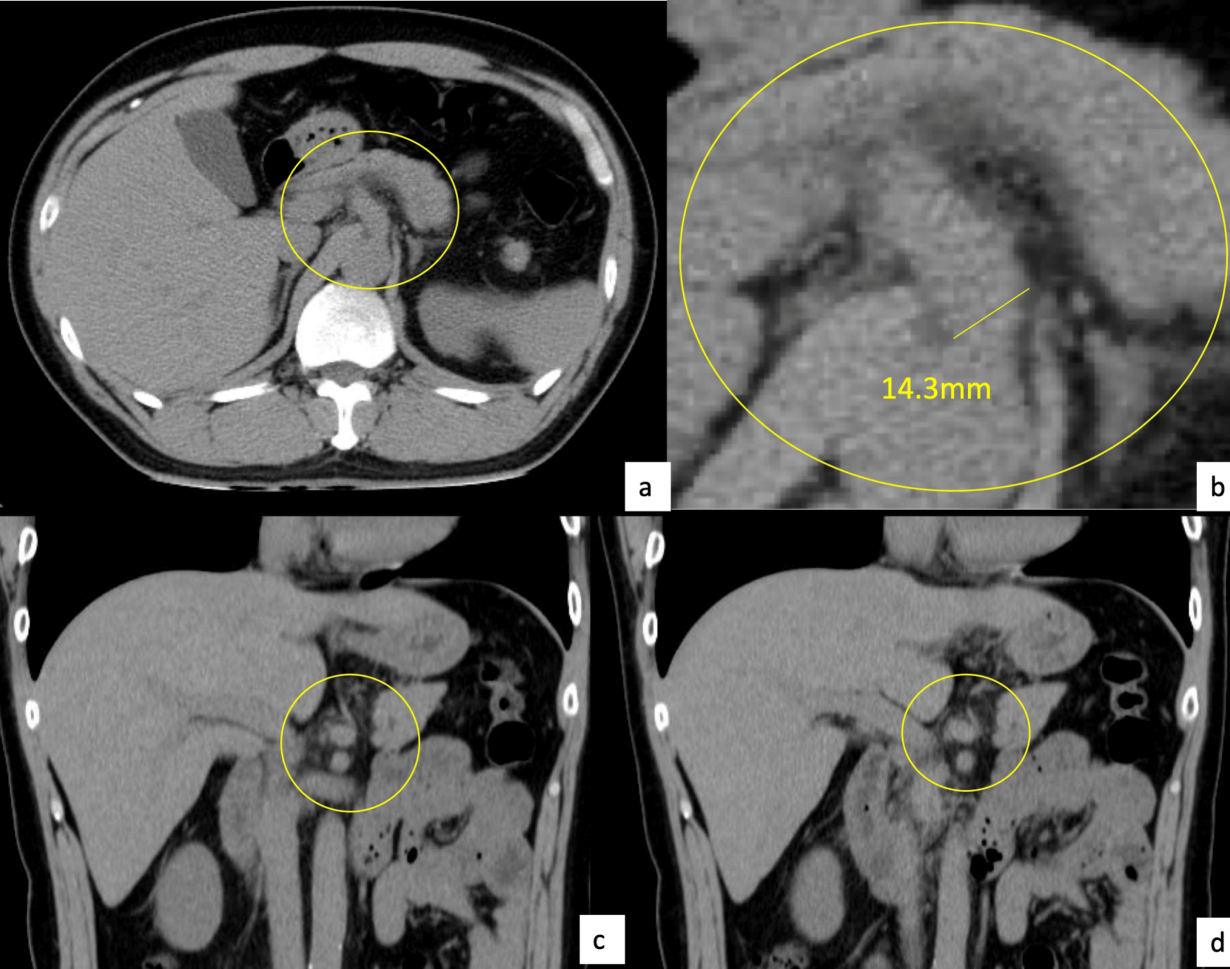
Abdominal non-contrast CT images Arterial dilatation of the celiac artery and opacification of the fatty tissue surrounding the celiac artery are observed (encircled)
